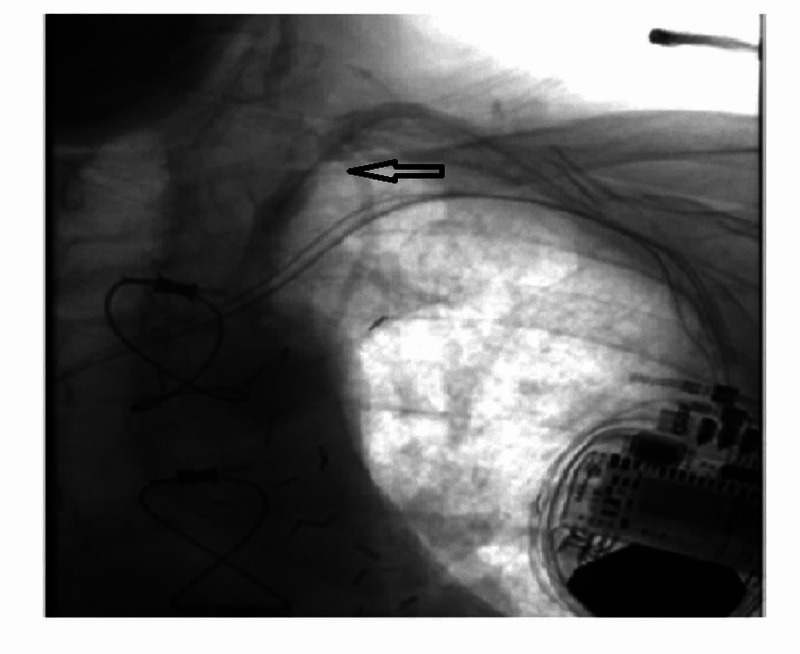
CT angiogram shows proximal left subclavian artery stenosis (arrow).
